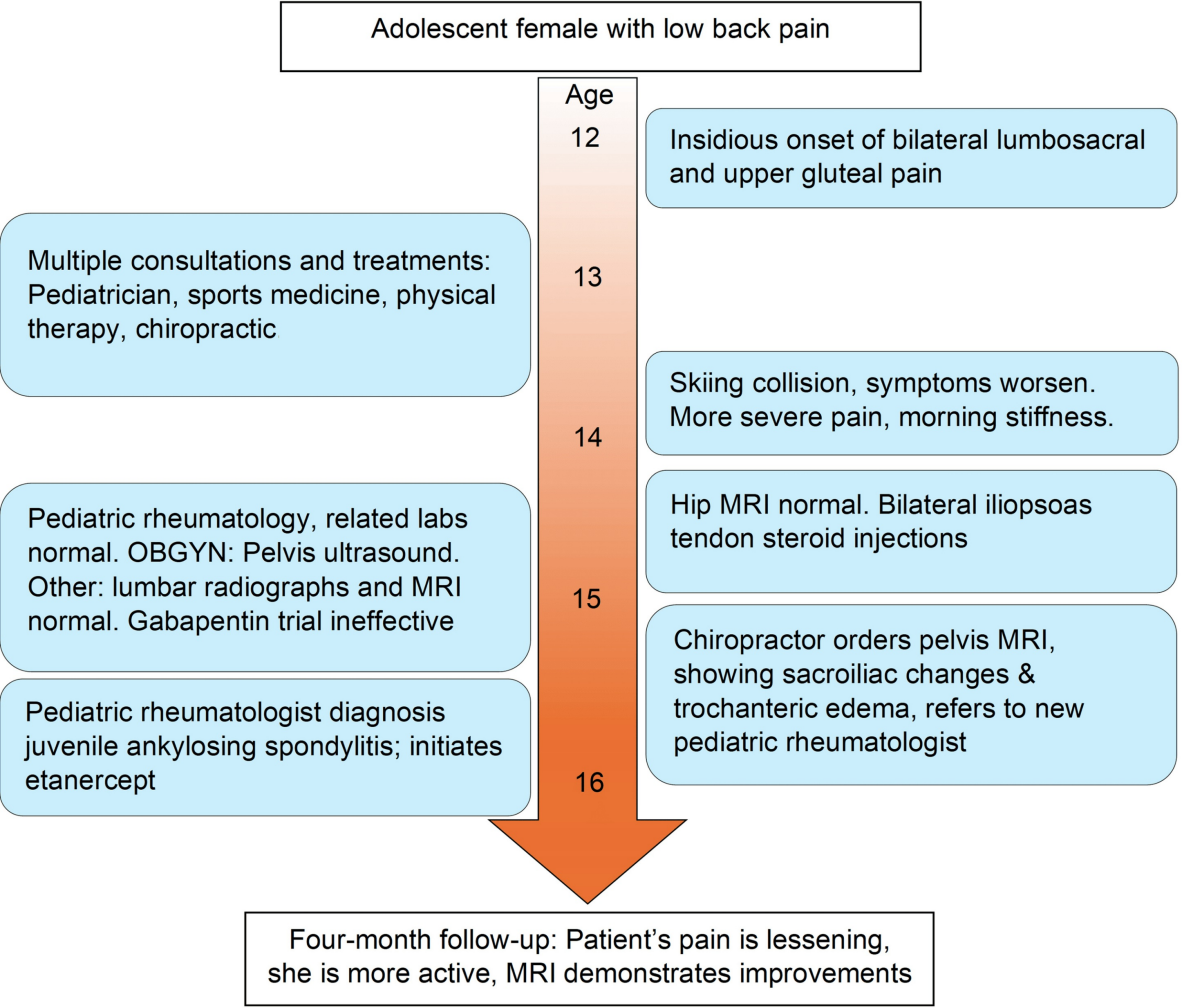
Timeline of the case. OBGYN: obstetrician-gynecologist; MRI: magnetic resonance imaging
